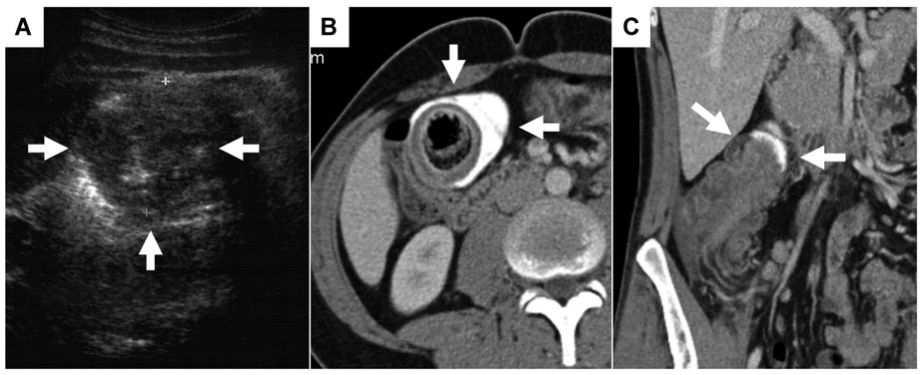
Pre-operative imaging of the abdomen by ultrasound and computertomography. A) Transabdominal 5MHz Ultrasound examination revealing a 47 mm diameter round mass in the right upper abdominal quadrant (arrows). The computed tomography of the abdomen in an axial plane (B) with coronal reconstruction (C) showing the cystic duplication with mucosal invagination in the ascending colon. Notice the rectal contrast filling that stops at the lesion (arrows).
